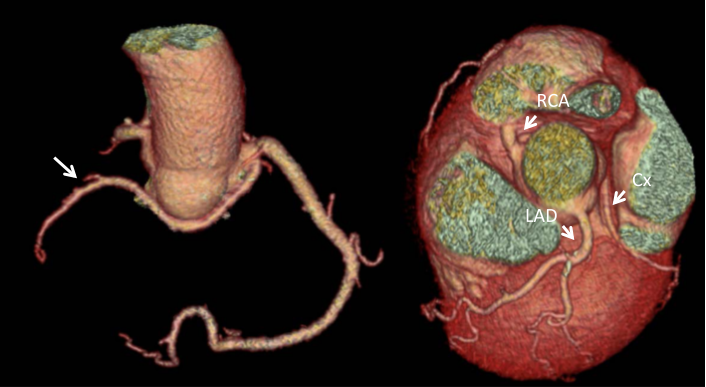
Volume-rendered three-dimensional reconstructions of the coronary tree and heart by CT coronary angiography in a patient presenting with previous syncope showing the circumflex artery arising from the right anterior sinus with anomalous retroaortic course.
